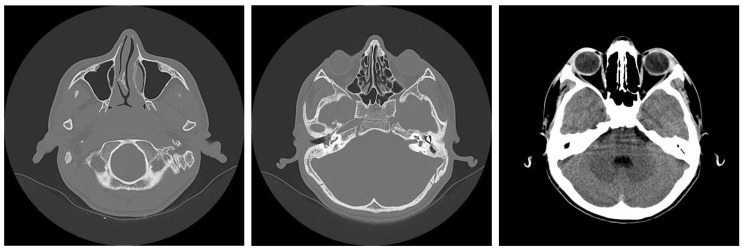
Patient with the diagnosis of right chronic suppurative polypous chronic polypous otomastoiditis, left chronic otomastoiditis, deviated nasal septum, and chronic hypertrophic rhinitis. CT examination, axial sections, bone, and parenchymal window—hypertrophy of bilateral middle nasal turbinates, accentuated on the left side; reduced pneumatization of mastoid cells on the left side; lack of air cell development in the mastoid cells; presence of effudion with increased densities in the right mastoid.
